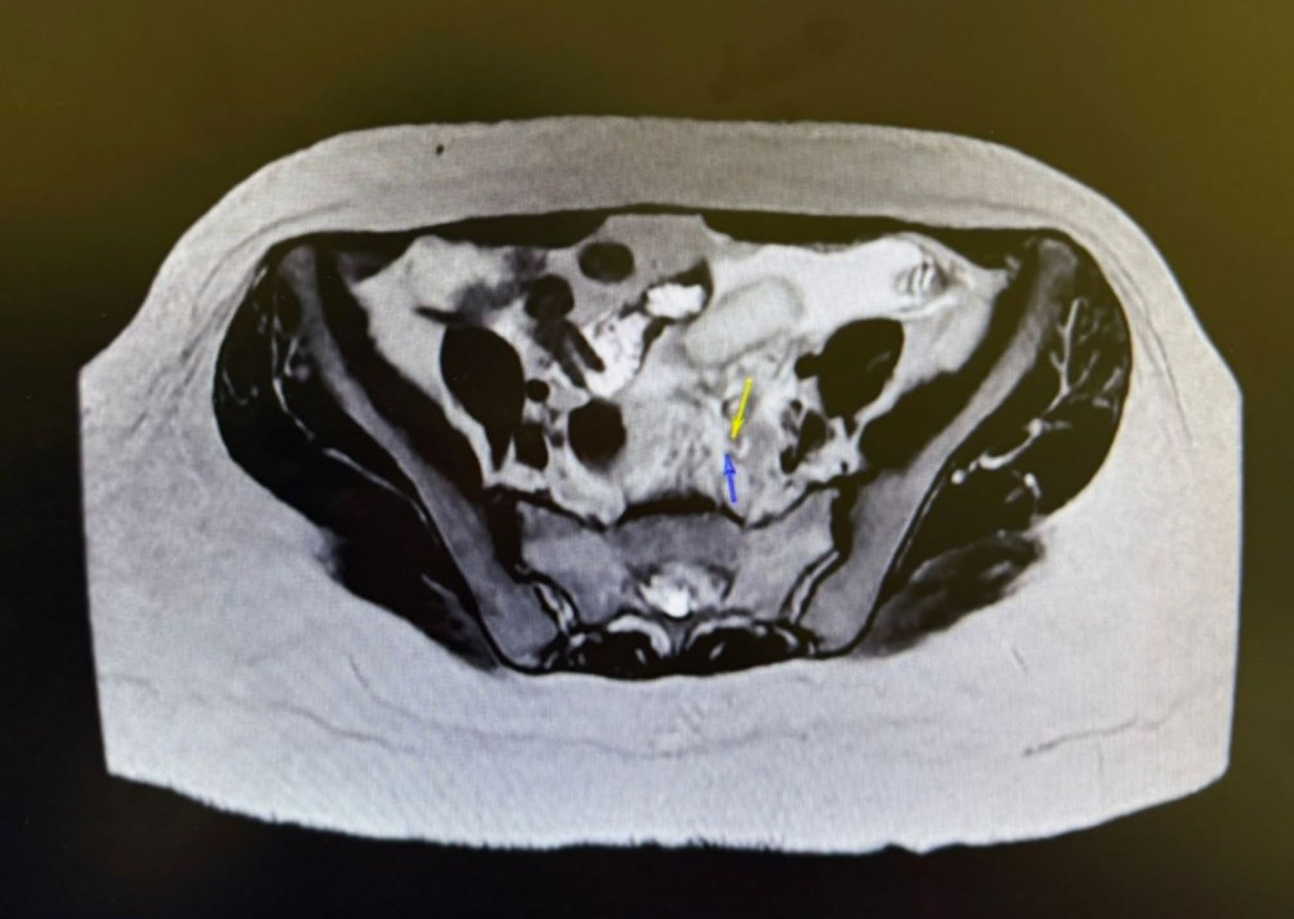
Axial cut MRI- demonstrating free fluid with a left-sided possible adaxial mass
